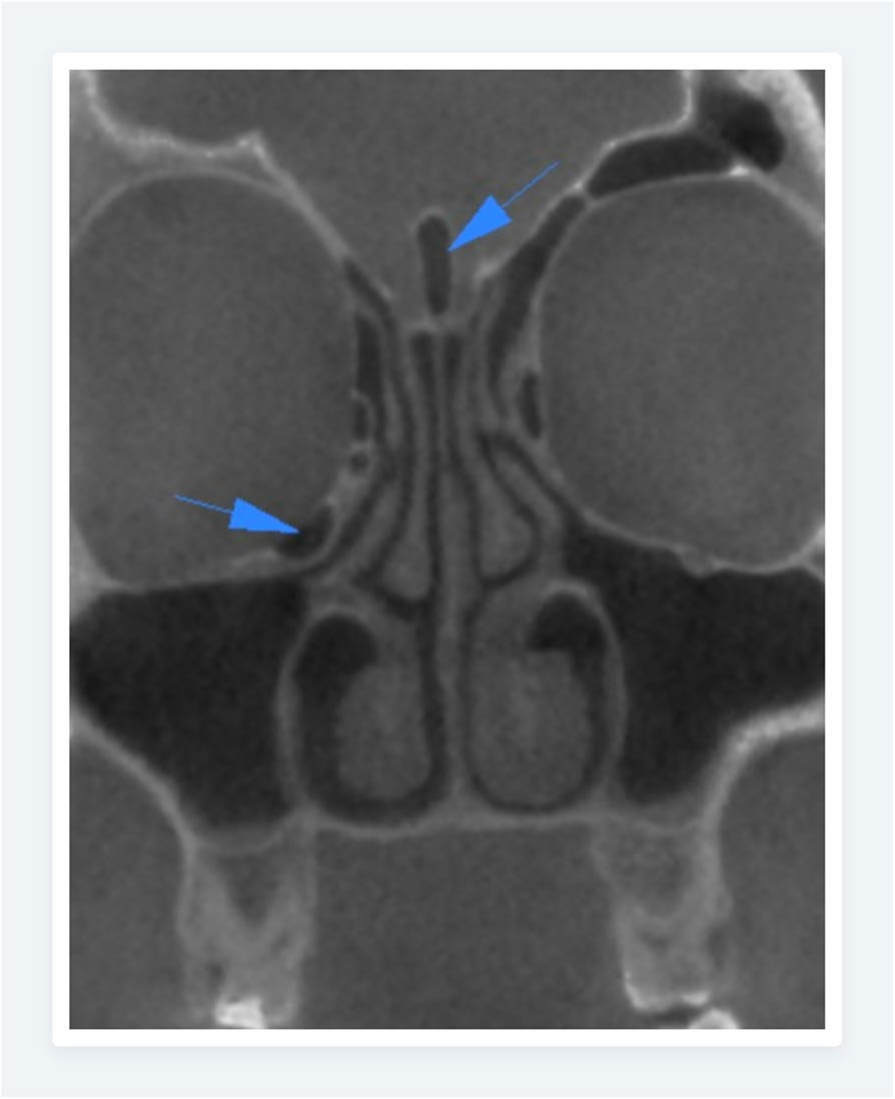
Coronal CBCT image of the Haller cell (on the right side) and crista Galli pneumatization with arrows
